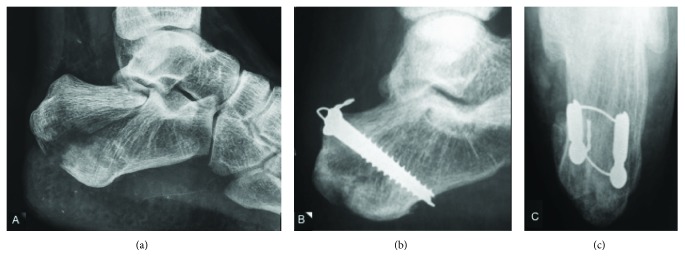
(a) Left lateral hindfoot radiograph shows an intra-articular calcaneal avulsion fracture occurring in conjunction with a fracture of the medial process (Beavis NC, Squires IV). ((b) and (c)) Lateral hindfoot and axial calcaneus radiographs show modified cerclage wire tension band technique through two 7.0 mm partially threaded cannulated screws. Observe the configuration of the wire on the axial radiograph.
